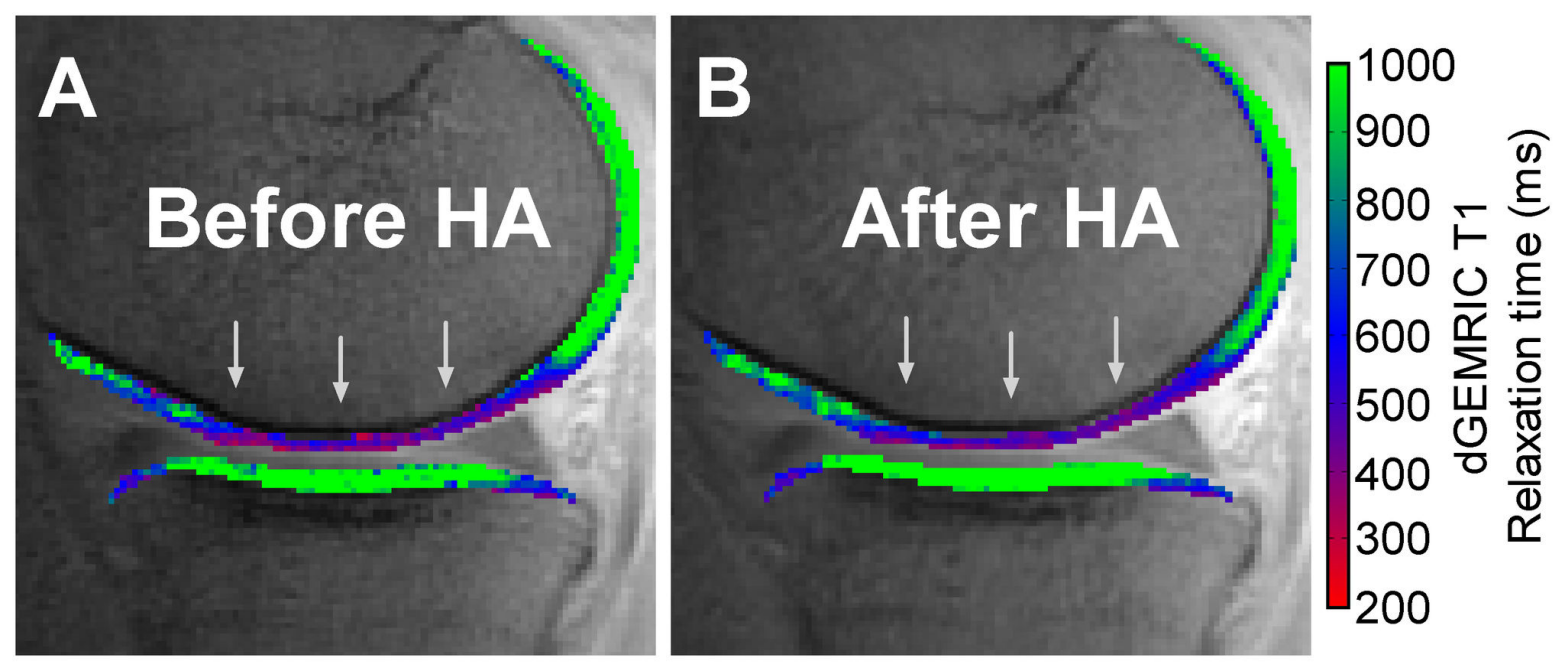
dGEMRIC color overlay representing cartilage sGAG content at baseline and follow-up. Representative sagittal central MR image through the medial tibiofemoral compartment of an early-stage OA knee before (A) and after (B) viscosupplementation with HA. The T1 color map of the cartilage clearly shows a region with relatively lower T1 relaxation times (grey arrows in A), indicating relatively lower sGAG content in the weight-bearing femoral cartilage before viscosupplementation (A). After HA injections (B), however, the region with relatively low T1 relaxation times is still present (grey arrows in B).
